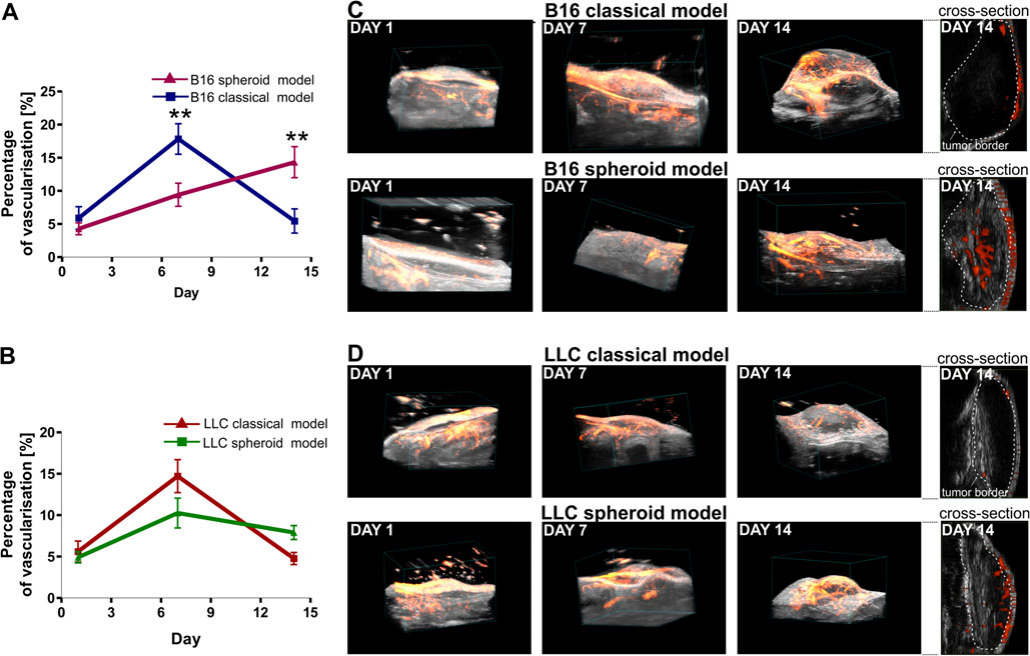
Development of tumor vasculature in spheroid-plug and classical model assessed by Doppler 3D-USG analysis. a Vascularization in B16 spheroid-plug and classical model tumors, observed at days 1, 7, and 14. b Vascularization in LLC tumors observed at days 1, 7, and 14. c, d Visualization of vessels in classical and spheroid-plug model. Two-way ANOVA with Bonferroni post-test (*p* < 0.01) between models in a given day
